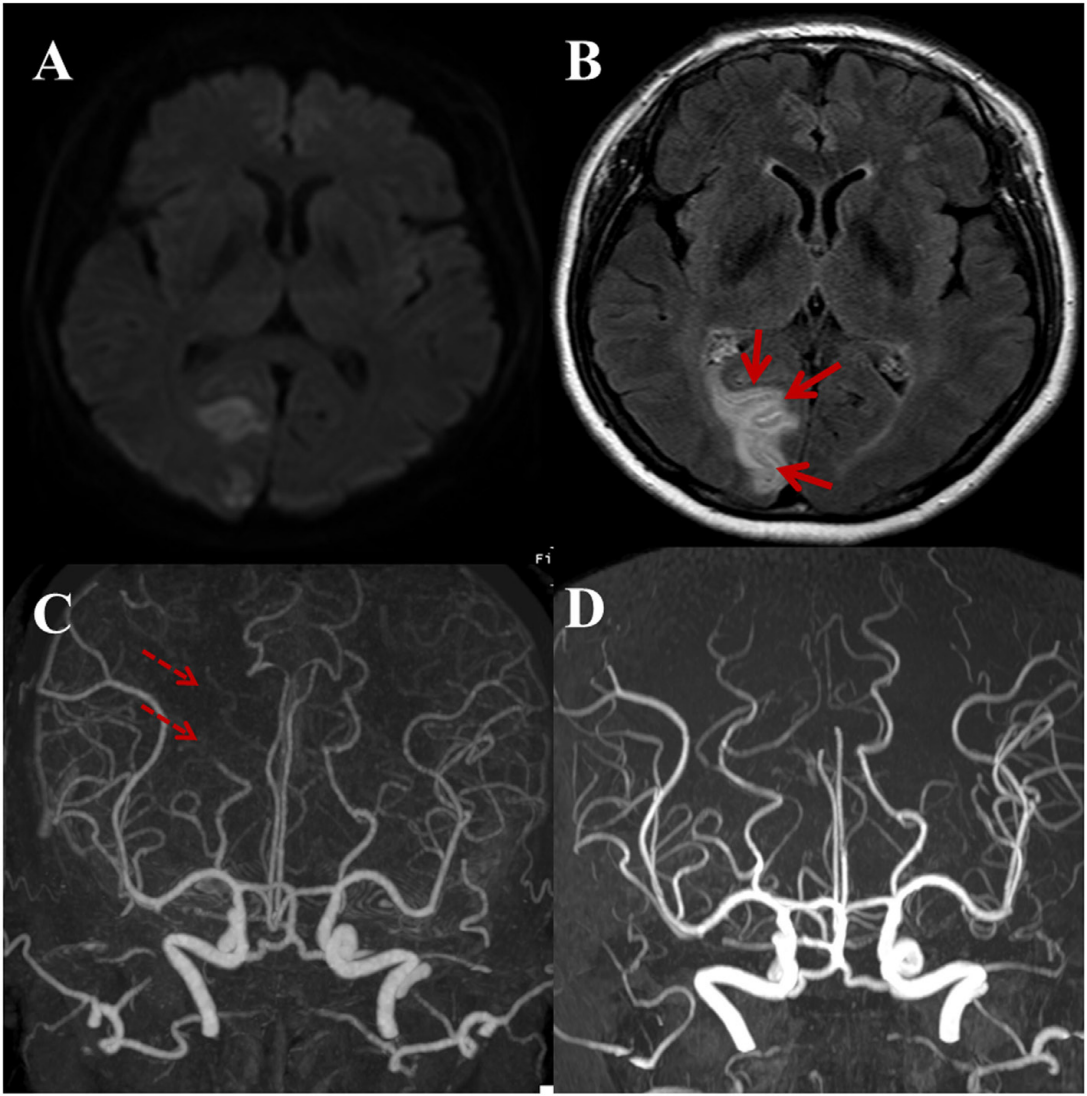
A 59-year-old male with a complaint of acute onset left side visual field defect in both eyes. Regional pattern of hyperintense acute reperfusion marker. (A) DWI shows acute infarction at the right occipital lobe involving the visual cortex. (B) Post-contrast fluid-attenuated inversion recovery image shows sulcal enhancement along the right occipital lobe (arrows). Compared with outside CTA (C), three-dimensional time-of-flight MRA (D) shows the recanalization state of right distal posterior cerebral artery (dashed arrows).
